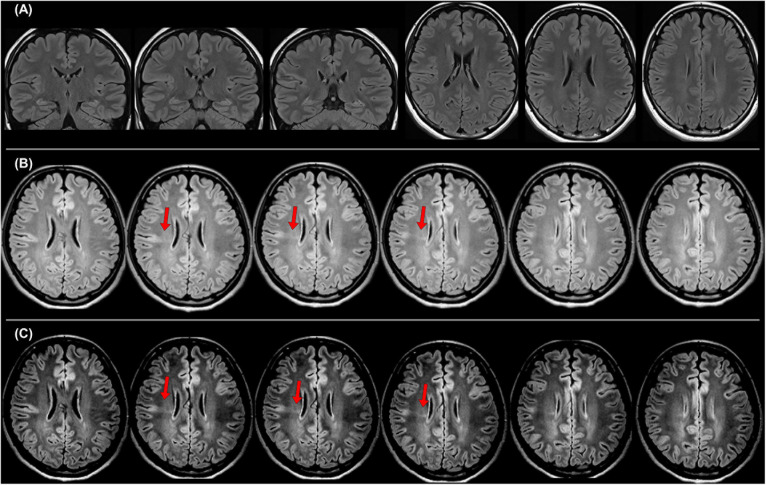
A case with drug-resistant focal epilepsy, who benefited from the official standard protocol for epilepsy. It was impossible to detect any abnormalities in both coronal and axial 2D fluid-attenuated inversion recovery (FLAIR) images with 3-mm slice thickness (A), but the 3D FLAIR images revealed findings of a bottom-of-sulcus-type focal cortical dysplasia with transmantle sign (B), and changing the signal range is sometimes helpful to clearly visualize the lesion (C). The pathological finding was focal cortical dysplasia type IIb.
